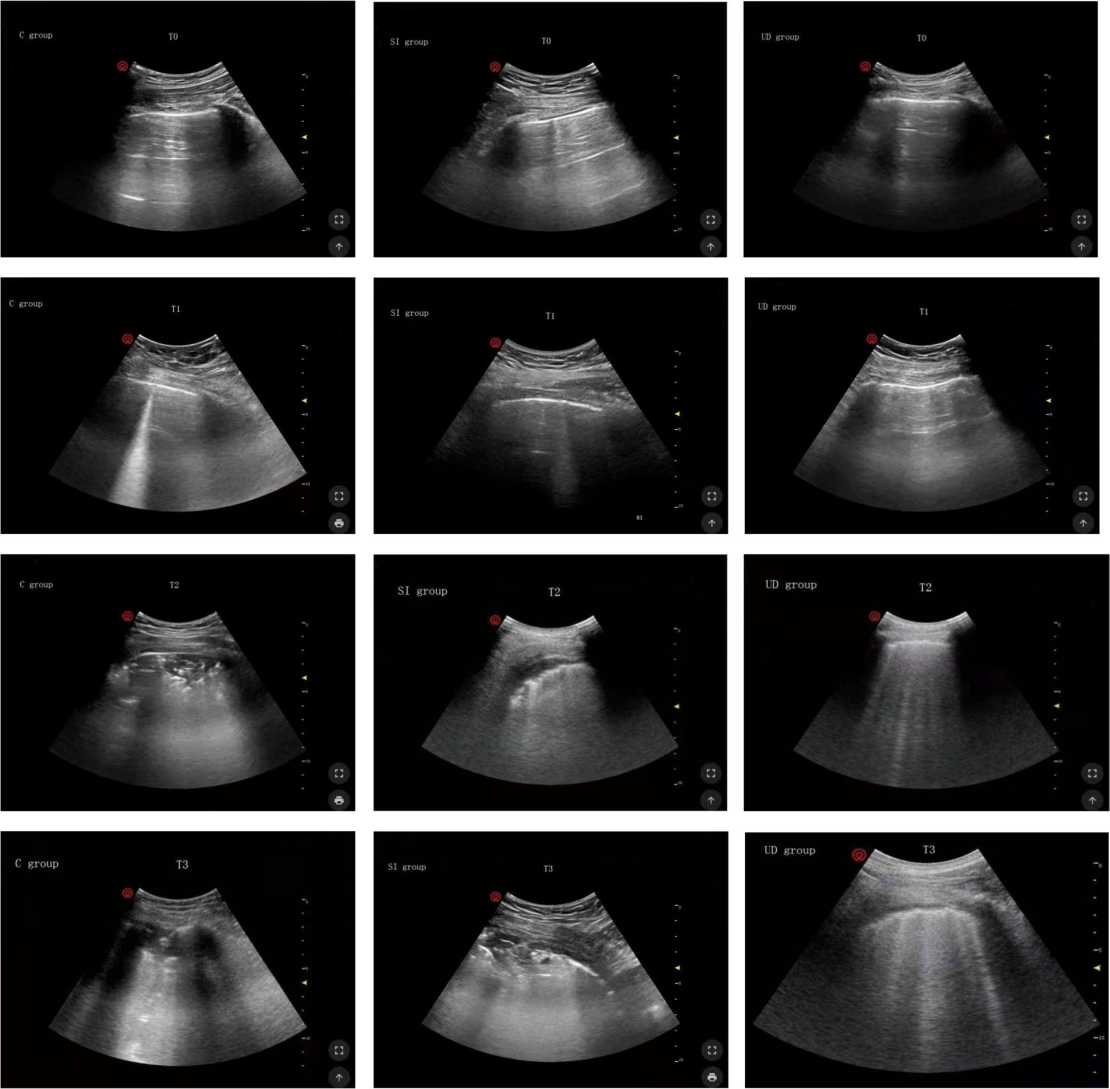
Lung ultrasound images of one representative patient in the lateral chest wall at different time points. T0: immediately before induction; T1: immediately after induction; T2: immediately after the end of surgery; T3: 30 min after tracheal extubation; C group: control group; SI group: sustained inflation recruitment manoeuvres group; UD group: ultrasound-guided recruitment manoeuvres group
